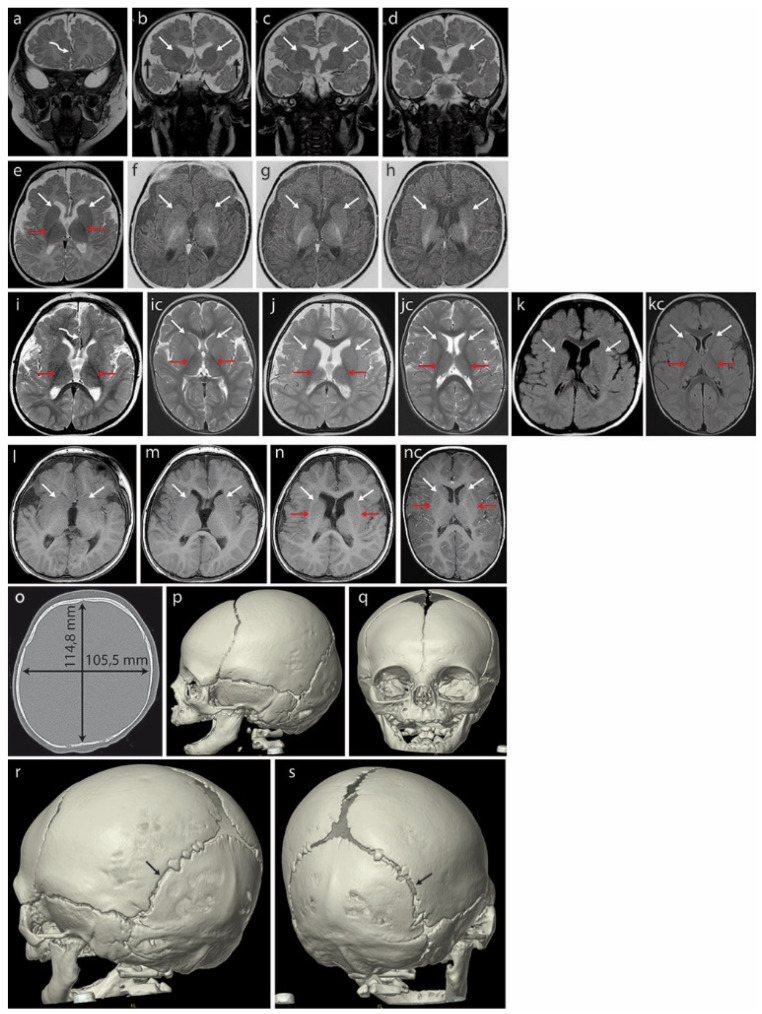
Brain MRI of the patient at 3 months (a–h) revealed hypoplasia/fenestration of the anterior falx cerebri with the gyri of the right cerebral hemisphere crossing the midline ((a)—curved arrow) and incomplete opercularization, which is normally complete at term ((b)—black arrows). Roundish basal ganglia in the coronal plane in T2-weighted images (T2WI) ((b–d)—white arrows). At this age, the posterior limbs of the internal capsules (PLICs—red arrows) are well myelinated: black in T2WI ((e)—axial plane) and white in the inversion recovery (IR) sequence, best depicting the myelinated structures (f–h). There is no trace of even the unmyelinated lines separating the caudate and lentiform nuclei that would represent the anterior limbs of the internal capsules (ALICs) ((b–h)—white arrows). A further brain MRI scan at 18 months (i–n) did not detect the ALICs which are normally appreciable at 10–11 months. The basal ganglia appear to be fused bilaterally in both hemispheres ((j–n) white arrows in the axial plane: (j)—T2WI, (k)—FLAIR, (l–n)—T1WI); opercularization remained incomplete (i). Normal PLICs ((i)—red arrows). (ic) Corresponding sections in the normal brain of an 18-month-old boy, T2WI. PLICs—red arrows; normal ALICs separating the caudate and lentiform nuclei—white arrows. (jc) Corresponding sections in the normal brain of an 18-month-old boy, T2WI. PLICs—red arrows; normal ALICs separating the caudate and lentiform nuclei—white arrows. (kc) Corresponding sections in the normal brain of an 18-month-old boy, FLAIR. PLICs—red arrows; normal ALICs separating the caudate and lentiform nuclei—white arrows. (nc) Corresponding sections in the normal brain of an 18-month-old boy, T1WI. PLICs—red arrows; normal ALICs separating the caudate and lentiform nuclei—white arrows. Computed tomography (CT) of the skull at age 4 months (o–s) showed a brachycephalic skull (the cephalic index of 91.9 exceeded the range of 76 to 81 compatible with a mesaticephalic skull in normal males). Asymmetric lambdoid suture: narrower on the left (r—black arrow) compared with the wider right one (s—black arrow).
